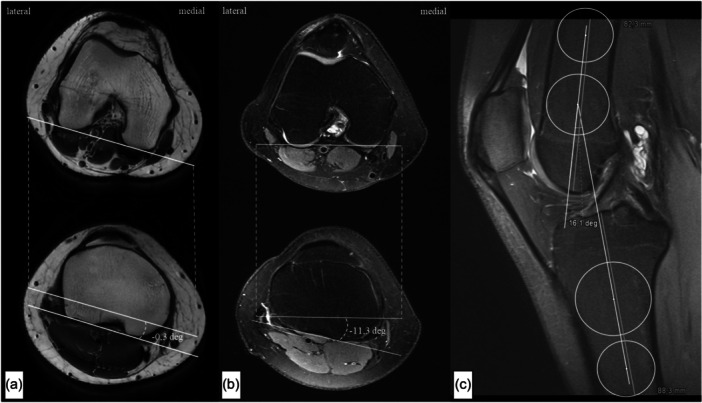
(a) iaTF rotation measurement in torsional MRI: Angle between DFC and DTH. (b) iaTF rotation measurement in routine MRI: Angle between DFC and DTH (c) Determination of femoral and tibial length in routine knee MRI and flexion measurement (the bony axes are defined by the centres of the circles). DFC, dorsal femoral tangent line (defined by the most posterior points of the femoral condyles); DTH, dorsal tibial tangent line (defined by the most posterior point of the proximal tibial plateau); iaTF, intraarticular tibiofemoral; MRI, magnetic resonance imaging [16, 34].
